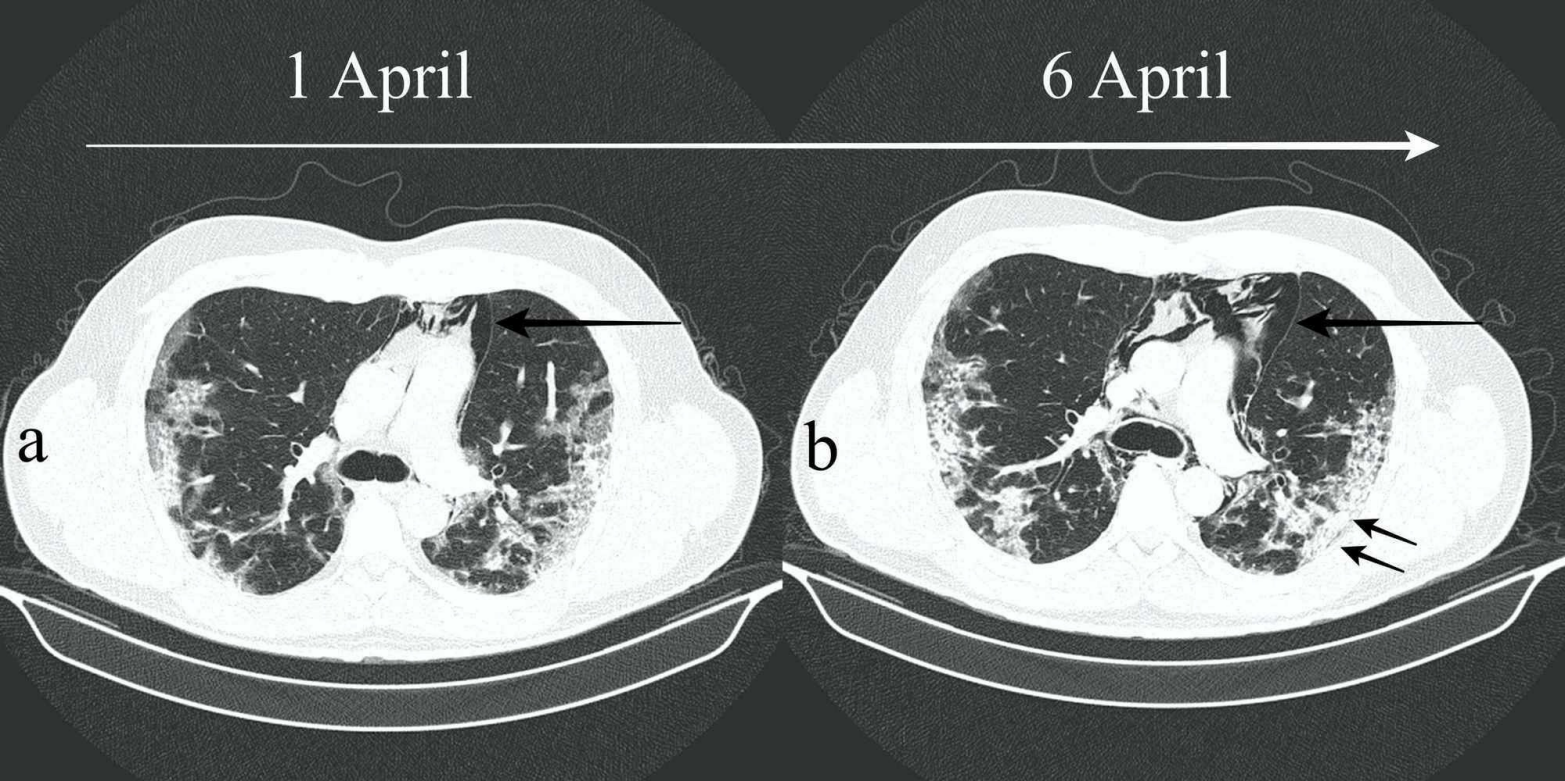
(a,b) Axial HRCT images showing progressive pneumomediastinum during the course of the novel disease (long arrows). In addition, previously noted ground-glass opacities started to progress into denser consolidations (short arrows). HRCT, high-resolution CT
